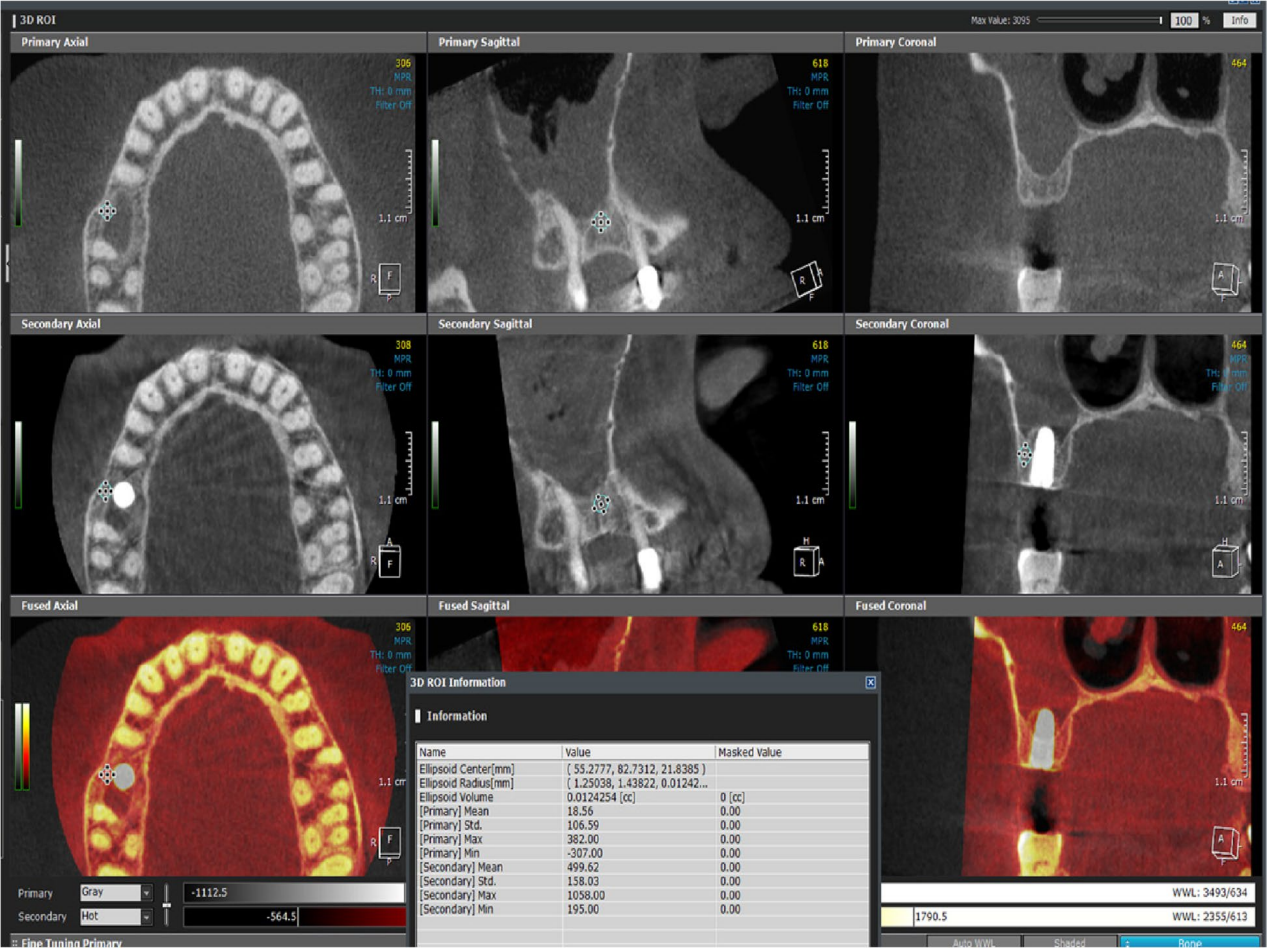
The ROI tool of the software was implemented to measure the bone density buccal and palatal to the implant site in the exact same place and with the exact area on the superimposed per-operative and post-operative CBCTs
